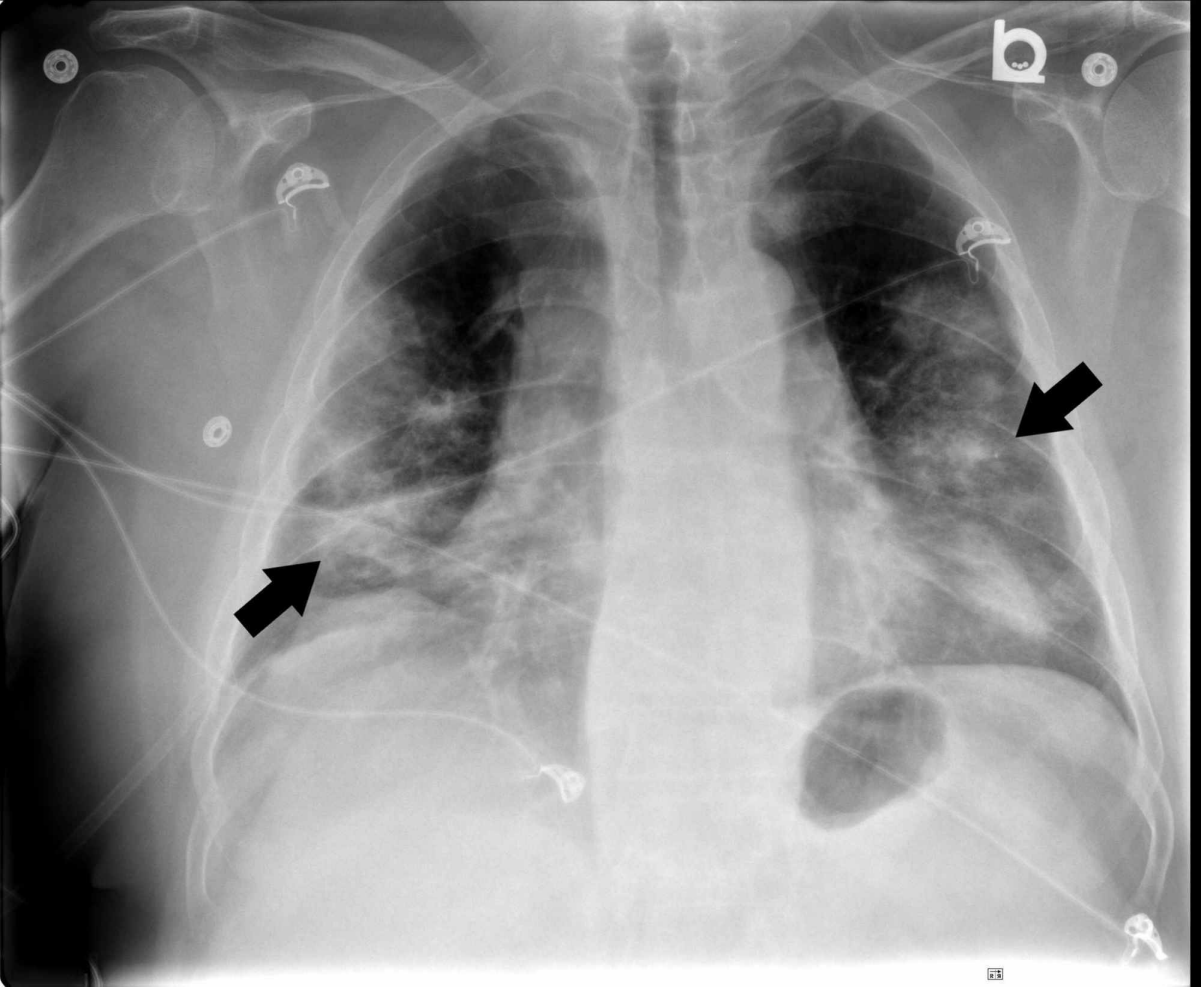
Chest radiograph on admission showing diffuse, bilateral air space opacities (arrows) in the mid and lower lung zones.
